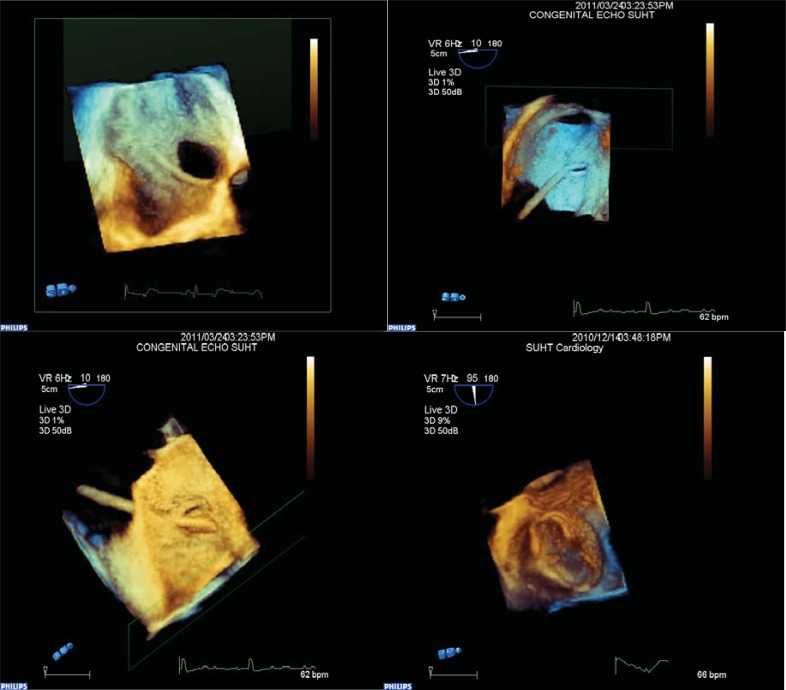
Images captured during real time three dimensional transoesophageal echocardiography for device closure of patent foramen ovale
